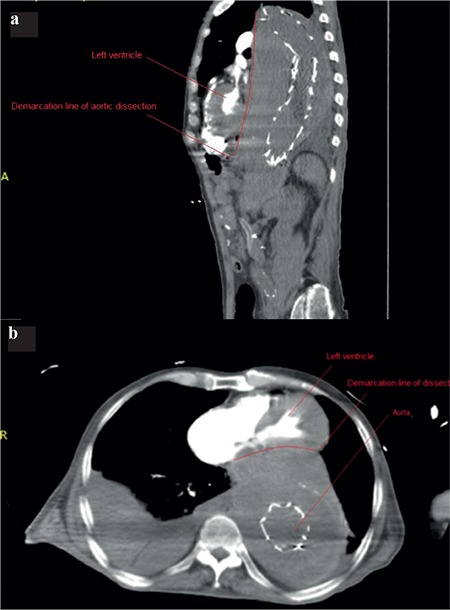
a-b. Sagittal computed tomography image showing the dissecting aortic aneurysm compressing the left heart chambers when the patient was admitted to the hospital (a), Transvers computed tomography image at the level of the ventricle showing the dissecting aortic aneurysm compressing the left heart chambers when the patient was admitted to the hospital (b).
